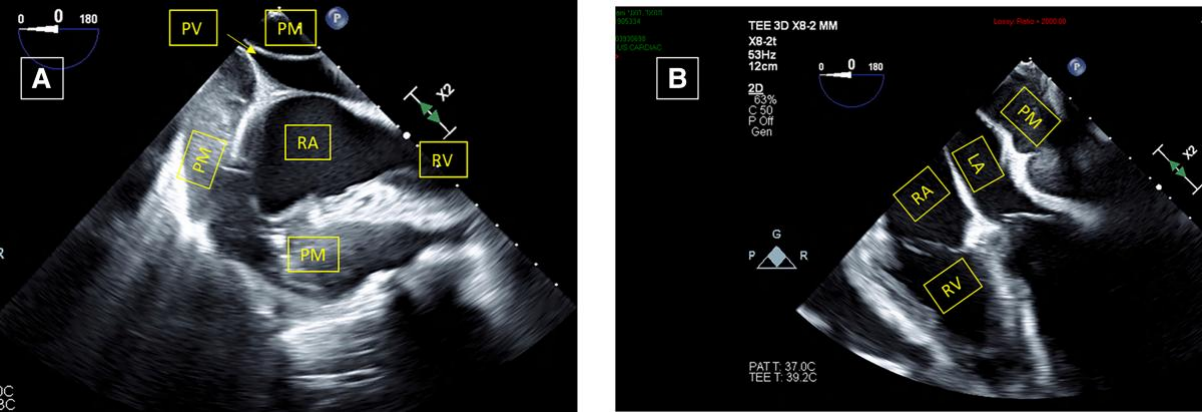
(*A*, *B*) Intraoperative transoesophageal echocardiography study showing large echogenic pericardial mass (PM) enwrapping right atrium (RA) and right ventricle (RV) and compressing right upper pulmonic vein (PV) and left atrium (LA).
